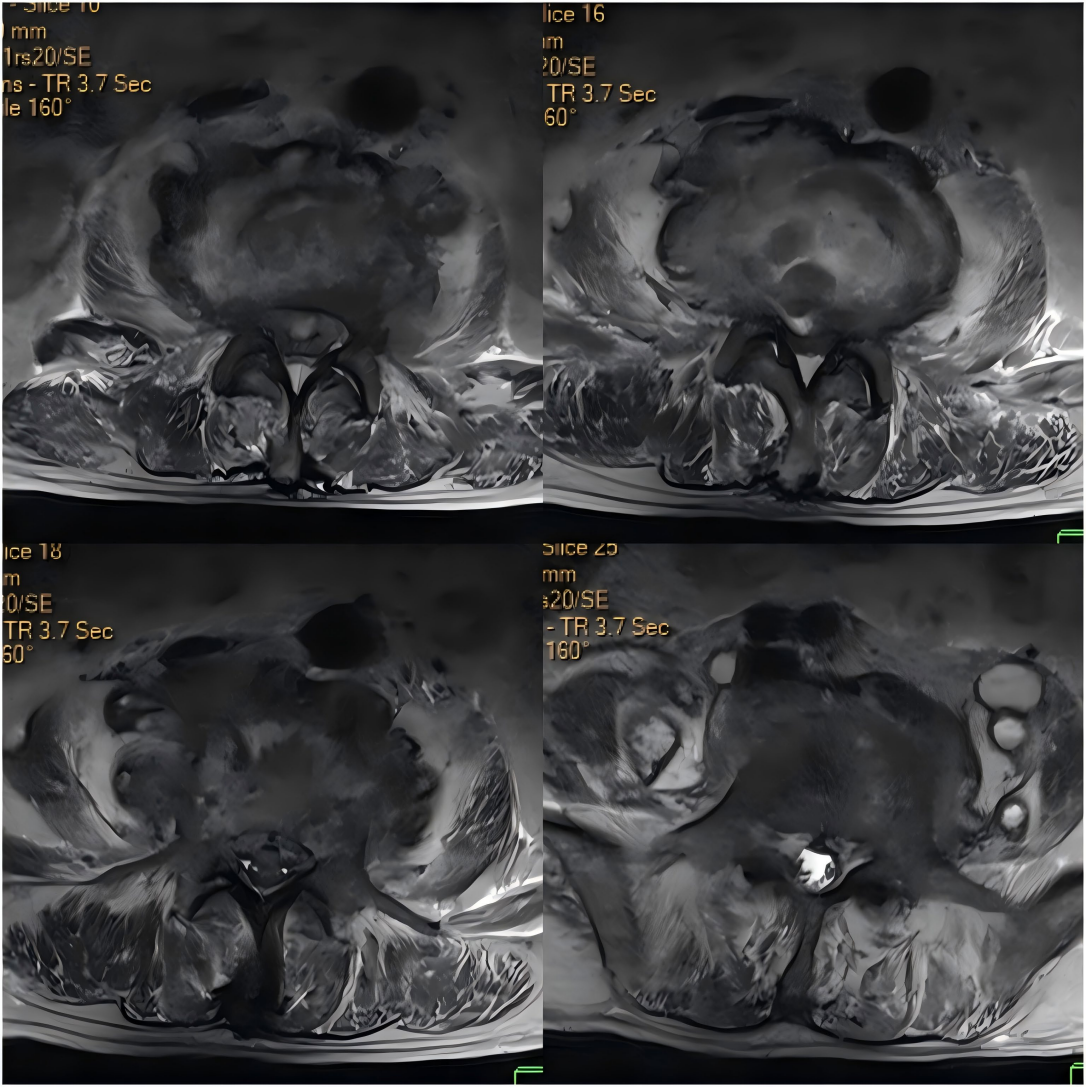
Axial contrast-enhanced CT image of the intervertebral discs.
